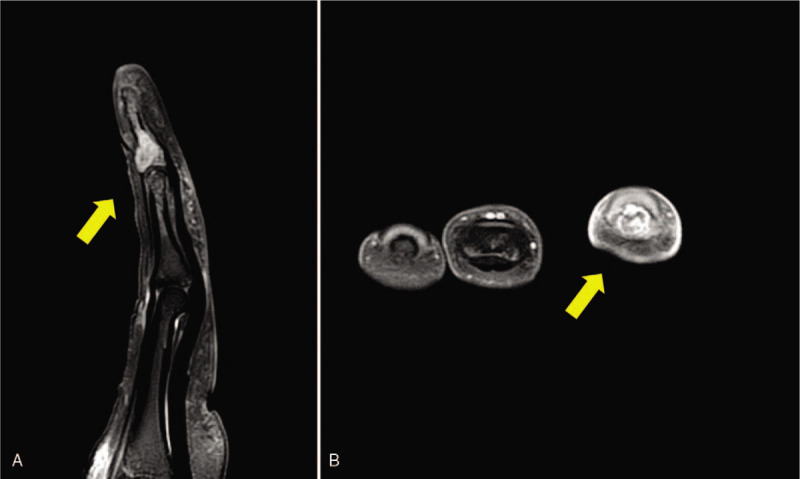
Magnetic resonance imaging demonstrating an eccentrically located mass that had expanded to the cortical bone.
